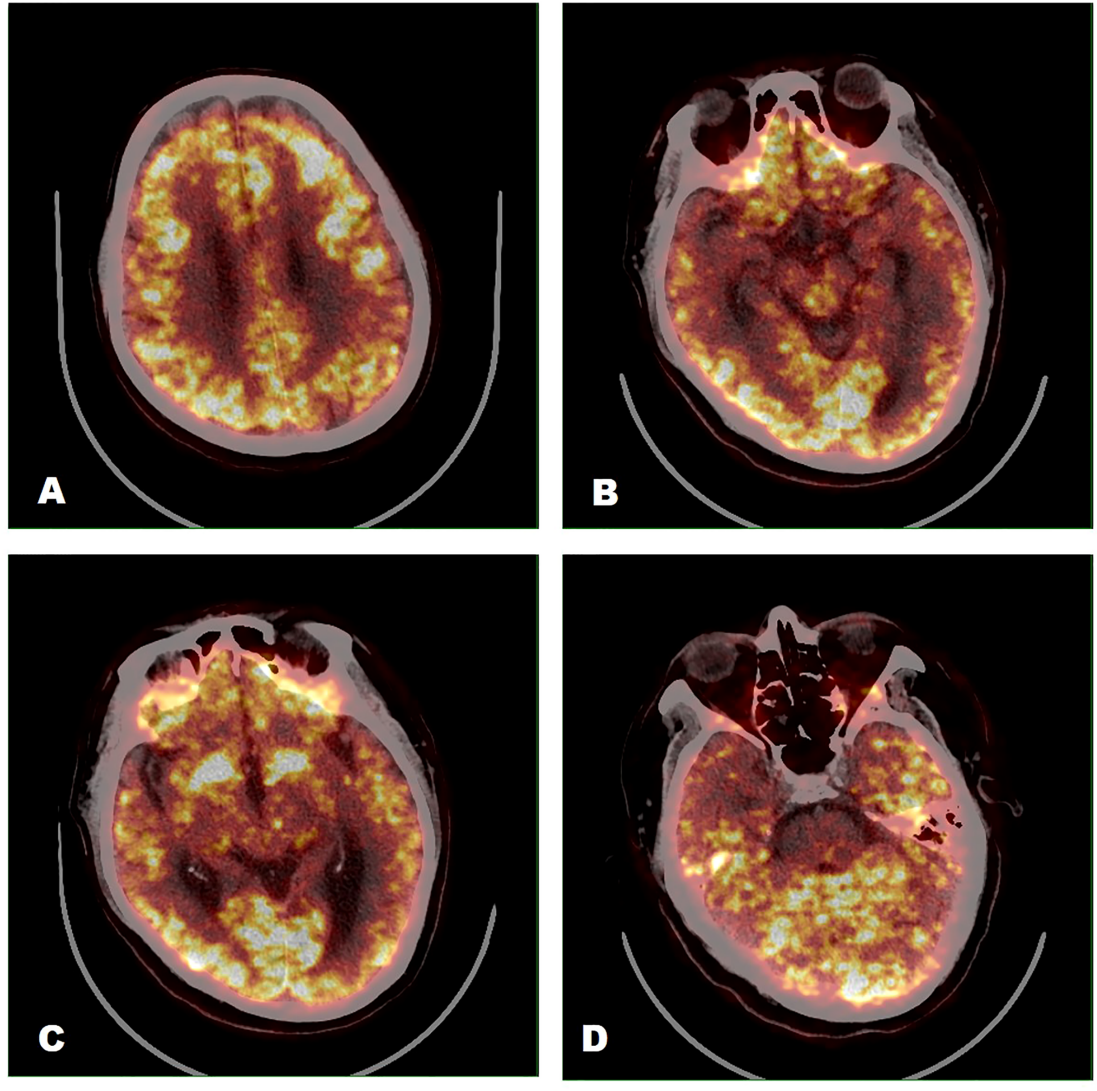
^18^F-FDG PET/CT image findings (Patient 18). Significantly lower FDG metadata levels were observed in the left parietal (A), occipital (C), parahippocampal gyrus (B, D), and dorsal thalamus (C).
